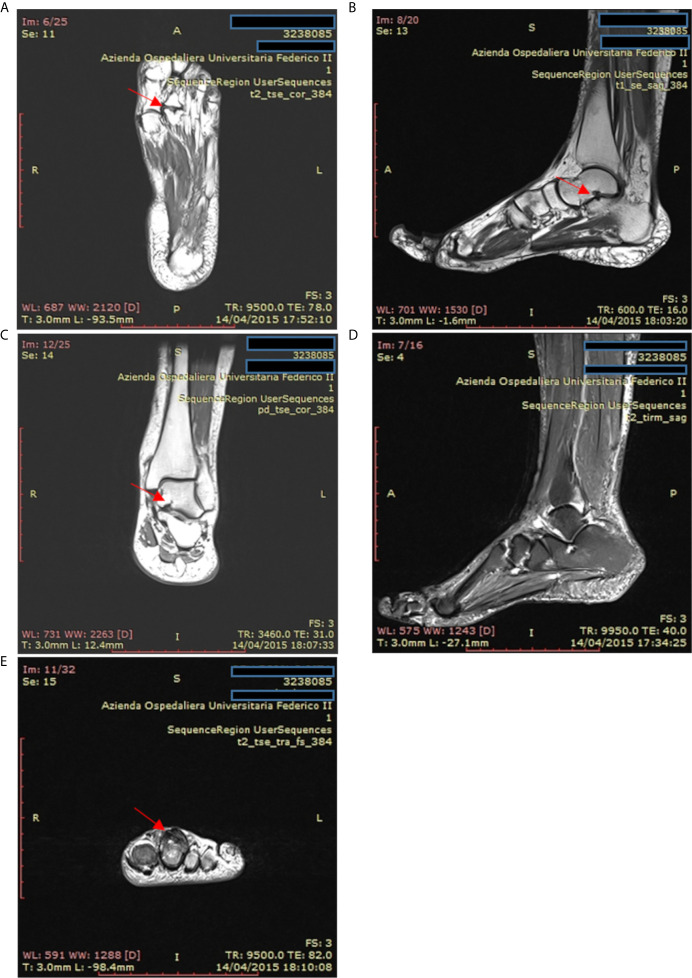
Magnetic resonance imaging of the ankle and foot in a patient with psoriatic arthritis and common variable immunodeficiency. (A) T2-weighted coronal magnetic resonance images of the left foot in a patient with psoriatic arthritis. Erosions are present at the second metatarsal-phalangeal joint (arrow). (B, C) T1-weighted sagittal axis and coronal magnetic resonance images showing cystic erosion (7 mm) of the floor of the sinus tarsi (arrow). (D) T2-weighted sagittal magnetic resonance images of foot and ankle showing joint effusion in the tibiotarsic compartment and enthesitis at the Achilles tendon insertion. (E) T2-weighted axial magnetic resonance images of the fingers from a patient with psoriatic arthritis exhibiting flexor and extensor tenosynovitis at the second finger (arrow).
